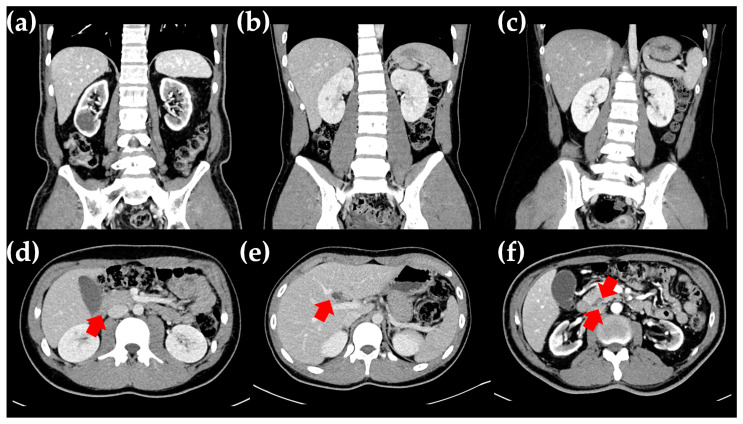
Radiologic findings in the proband and his family. (a–c) Abdominal CT findings show renal cysts in the proband’s father (a), the proband (b), and the proband’s sister (c). (d–f) The cortical thinning of both kidneys and atrophic changes in the pancreas (d) were found in the proband’s father, while a hepatic cyst (e) and agenesis of the dorsal pancreas (f) were found in the proband. The tail of the pancreas was not visible on consecutive axial slices, showing only the head and body of the pancreas (indicated by red arrow).
